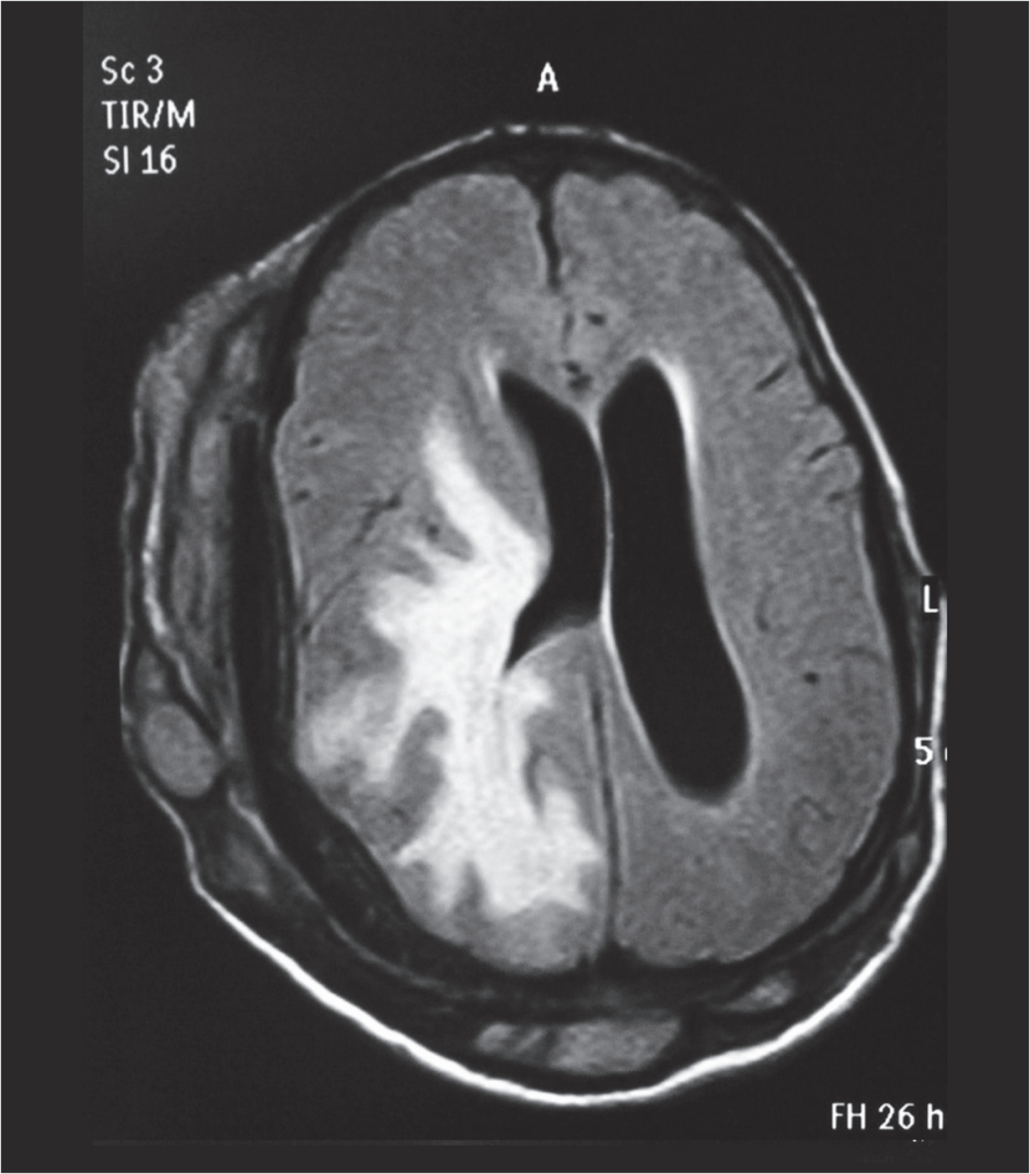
Cranial MRI showing generalised skin, subcutaneous tissue and bone involvement with massive intracranial spread.
